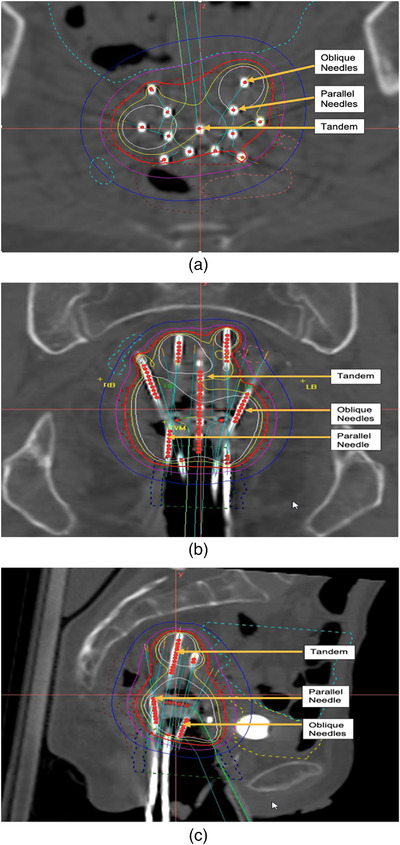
(a) Axial, (b) coronal, and (c) sagittal, CT view of Venezia applicator with needle orientation during treatment showing dose distribution by tandem, parallel and oblique needles
